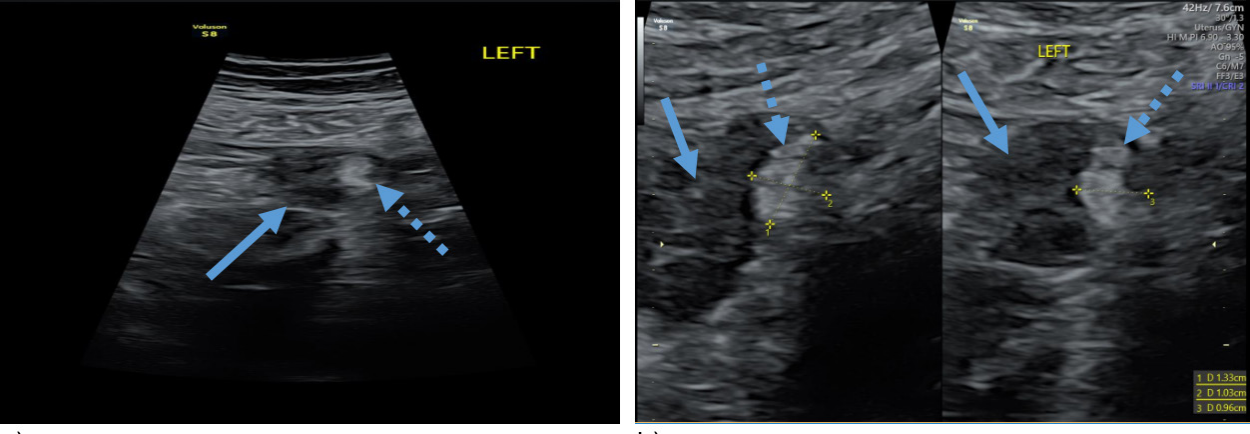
Transabdominal ultrasound revealing left ovary with a teratoma, (a) left ovary (arrow) with teratoma (dotted arrow), (b) measurement of a teratoma (dotted arrow) in the left ovary (arrow).
